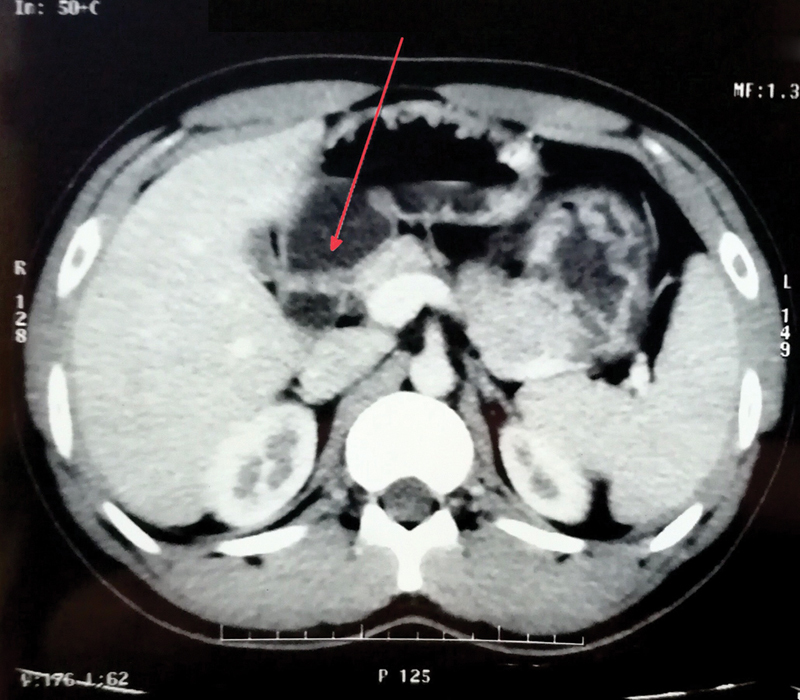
Computed tomography scan showing (red arrow) a polyp arising from second part of duodenum, ∼4 cm × 2 cm with no significant adenopathy.
